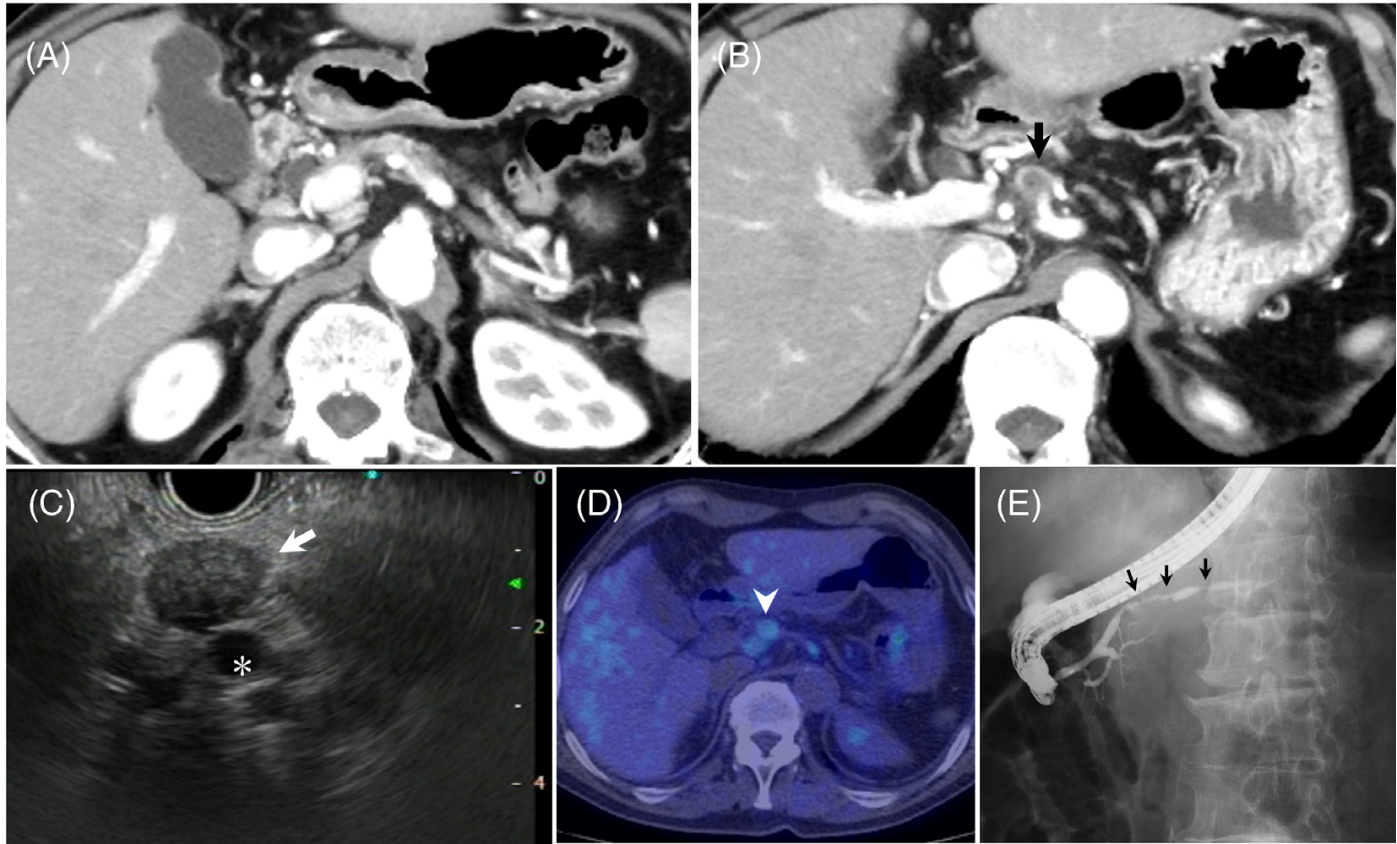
Imaging findings after 18 courses of FOLFIRINOX. (A) Abdominal enhanced computed tomography (CT) showing no tumor in the pancreas. (B) The tumor size is decreased to 15 mm, and it is located at the superior portion of the common hepatic artery (CHA; *arrow*). (C) Endoscopic ultrasonography showing a hypoechoic mass (*arrow*) only around the CHA (*). (D) [^18^F] fluorodeoxyglucose (FDG) positron emission tomography‐CT showing decreased FDG uptake (maximum standardized uptake value: 2.7) in the shrunken tumor (*arrowhead*) (E) Endoscopic retrograde pancreatography showing a difference in the diameter of the main pancreatic duct between the pancreatic body and tail (*arrows*). FOLFIRINOX: 5‐fluorouracil, leucovorin, irinotecan, and oxaliplatin
